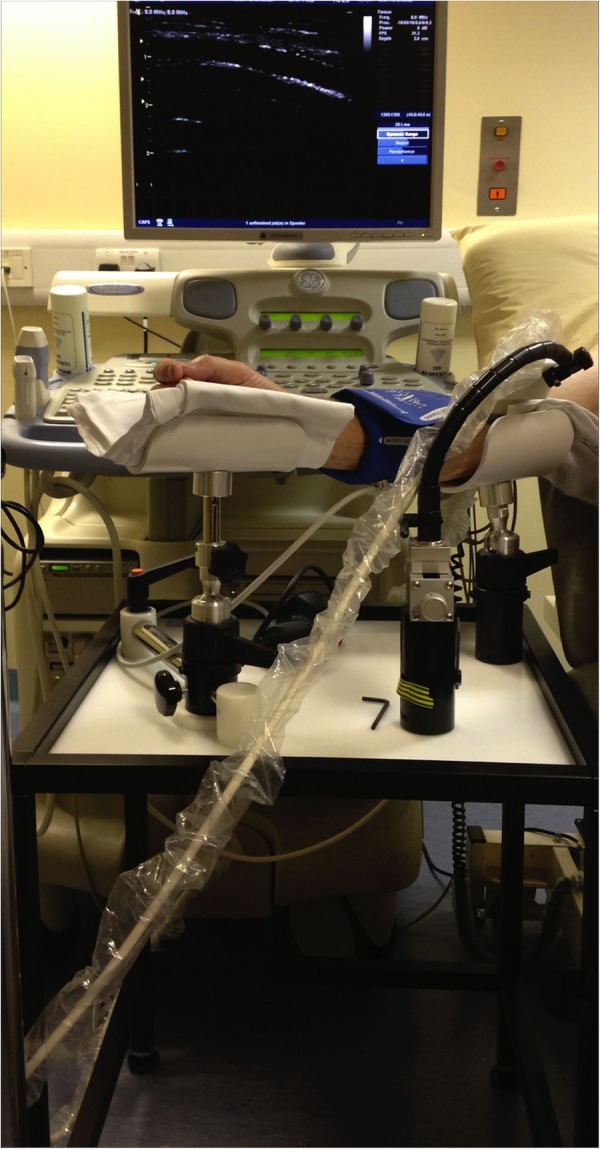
Custom built rig supporting the right upper arm and forearm, enabling the arm to be splinted in the anatomical position (elbow extended and forearm supinated), the ultrasound (USS) probe positioned proximal to the elbow and the sphygmomanometer cuff positioned distal to the elbow.
